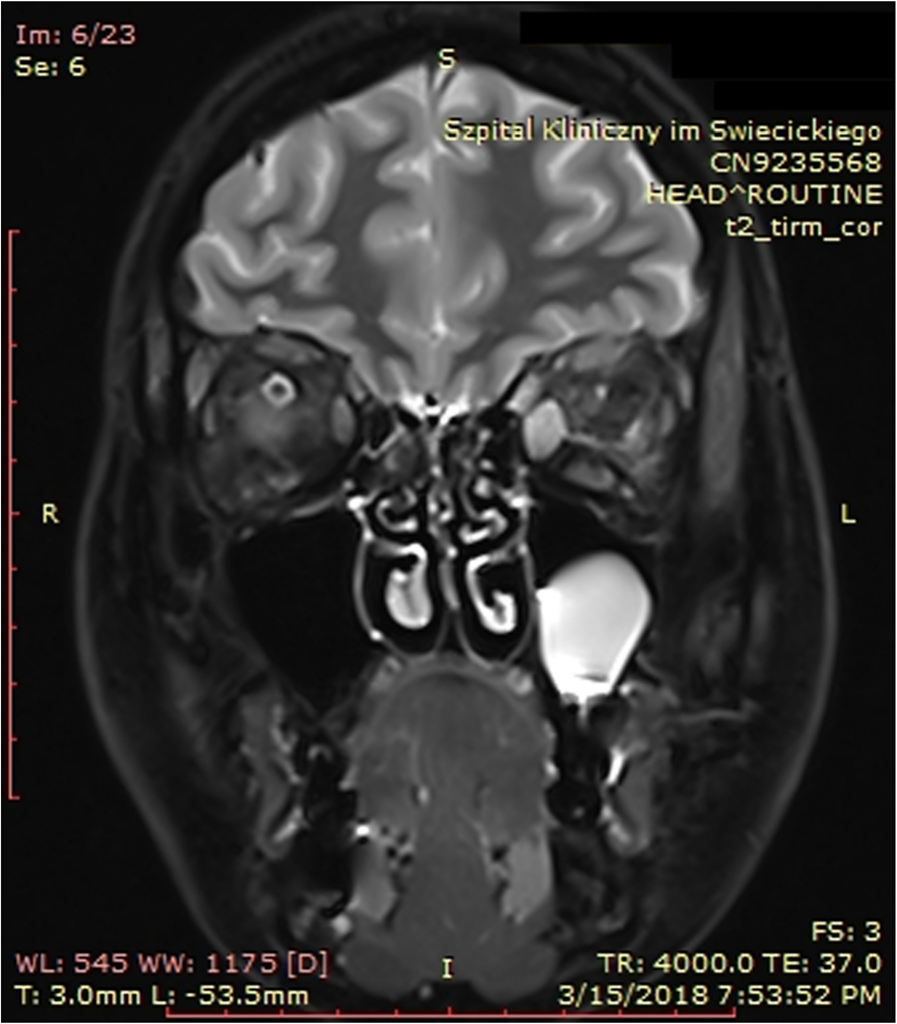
Increased T2 signal intensity from the left medial rectus muscle on MRI in a coronal plane
